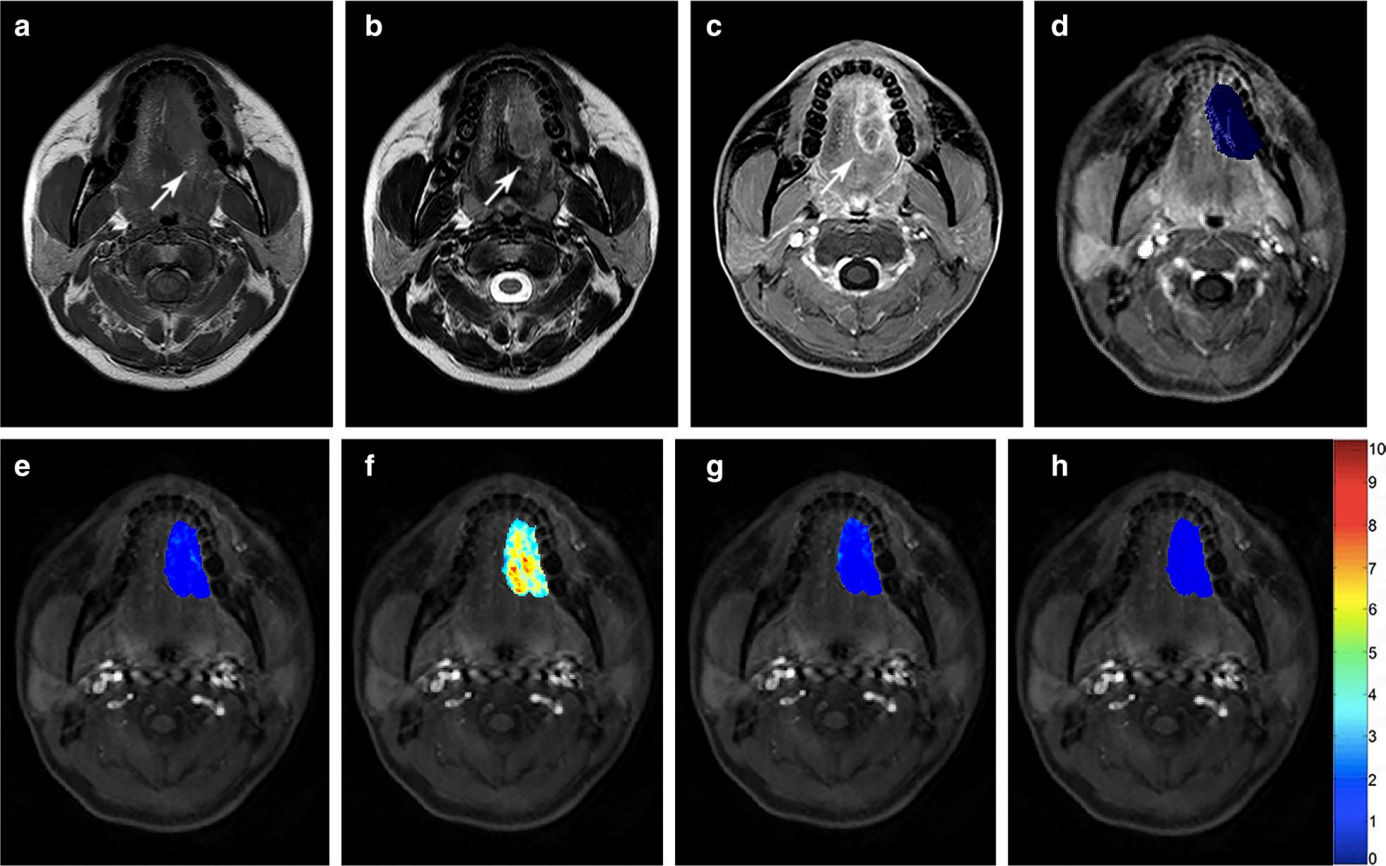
A well differentiated squamous cell carcinoma in the tongue, T2N2M0 (stage IV). a–c The tumor was seen as a 38 mm × 23 mm × 17 mm mass (arrows) with isointense signal on T1WI (a) and hyperintense signal on T2WI (b), with heterogeneous enhancement on fat-suppressed contrast-enhanced T1WI (c). d DCE-MRI parameters are evaluated by a 3-D VOI (arrow). e–h Pseudocolorized maps show individual parameters derived from DCE-MRI. The measured K^trans^ (e) is 0.043 min^−1^, K_ep_ (f) is 0.503 min^−1^, V_e_ (g) is 0.090, and V_p_ (h) is 0.009
